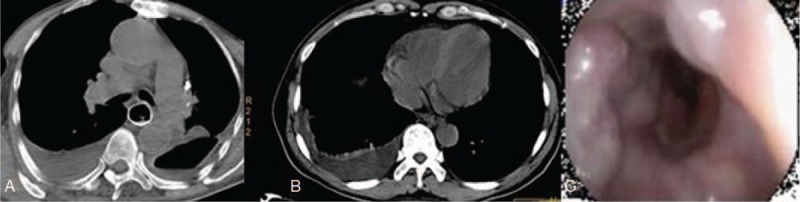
(A) CT image of the thorax showing a self-expandable metallic stent. (B) CT images showing complete closure of the esophagopleural fistula after stent removal. (C) Endoscopy images showing complete closure of the esophagopleural fistula 6 months later.
